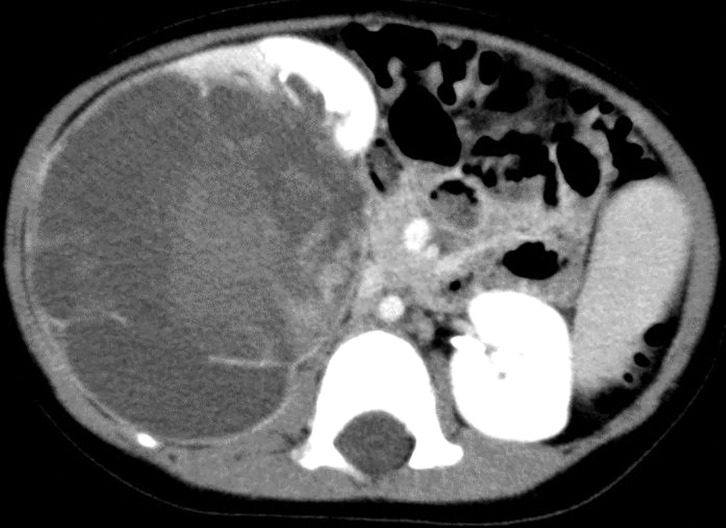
Contrast enhanced computed tomography of the abdomen and pelvis. A 10-cm multicystic tumor with a solid component is detected in the right kidney.
